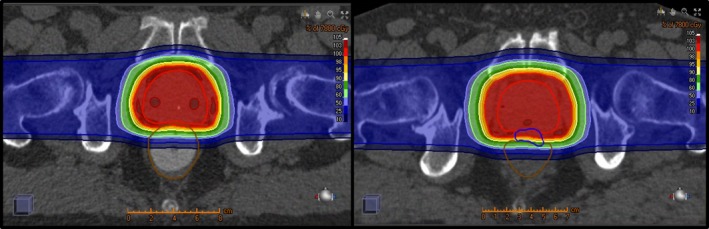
Left: CT of a prostate patient with an endorectal balloon (ERB). Right: CT of a prostate patient with a hydrogel spacer (GEL). Dose shown to a prescription of 78 Gy_RBE_.
